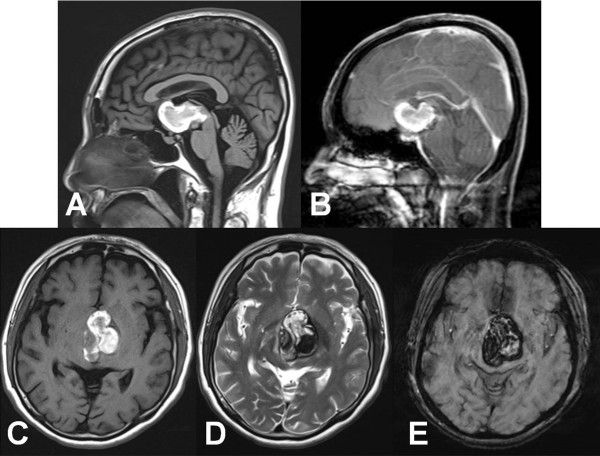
Preoperative MRI images. Sagittal T_1_-weighted (A) and post-contrast T_1_-weighted (B) images show a non-enhancing hemorrhagic mass in the anterior third ventricle and hypothalamic area. Axial T_1_-weighted (C), T_2_-weighted (D), and susceptibility weighted (E) images demonstrate a typical cavernous malformation with heterogeneous signal intensity and hemosiderin rim indicating mixed acute or subacute stage hemorrhage.
